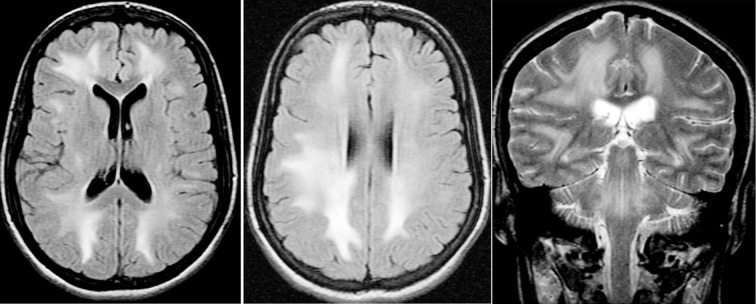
20 year SLE pt. with seizures. Axial FLAIR (A and B) and coronal T2 weighted images show extensive bilateral areas of increased signal intensity in cerebral white matter and brain stem.
